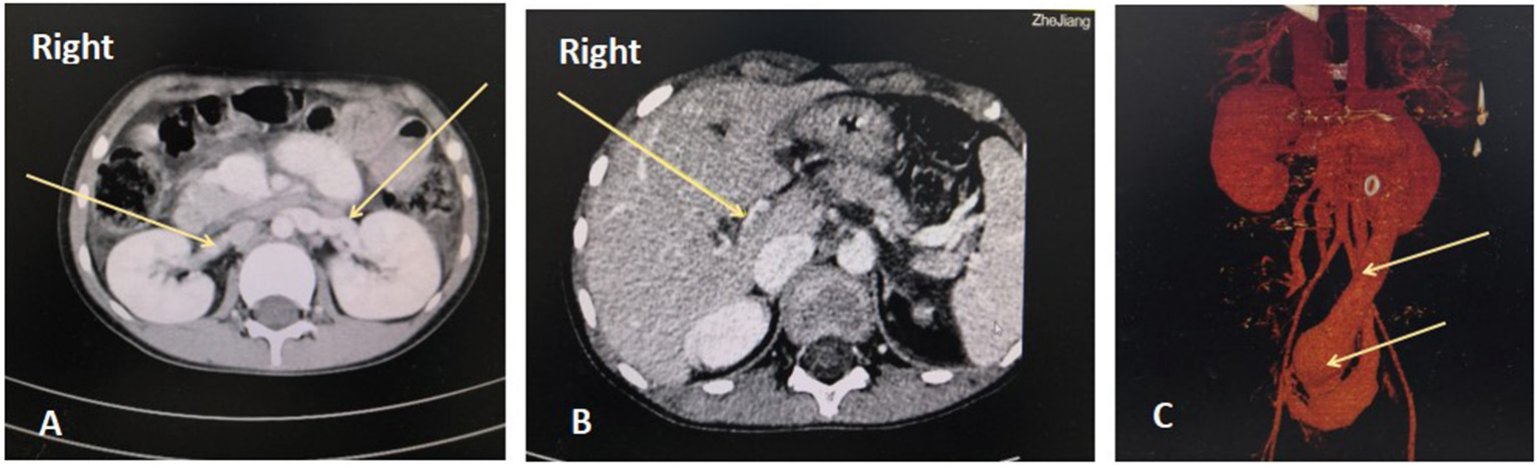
Abdominal enhanced computed tomography angiography (CTA). A. Bilateral renal veins are enlarged and thickened. B. The right branch of the portal vein is extremely thin, with a diameter of 1.2 mm. The left branch and the junction are not apparent in the images. C. The inferior vena cava and pelvic vein are remarkably dilated, and the widest point is 41 mm.
